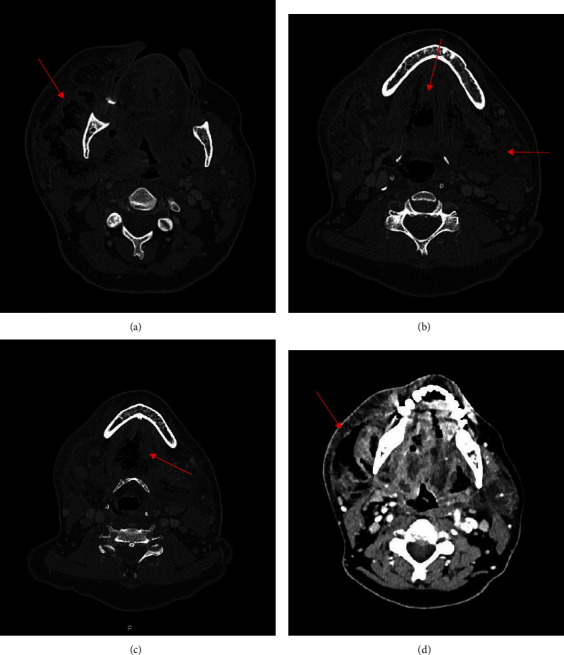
(a–d) Initial CT of the neck (transverse plane) highlighting the presence of gas bubbles (red arrows): at the right parotid gland posteriorly (a); oral floor extending up to the contralateral parotid capsule (b); sternocleidomastoid muscle medially and the thyroid space inferiorly (c); at the right parotid gland in a different cutting plane height (d) (13/02/2021).
